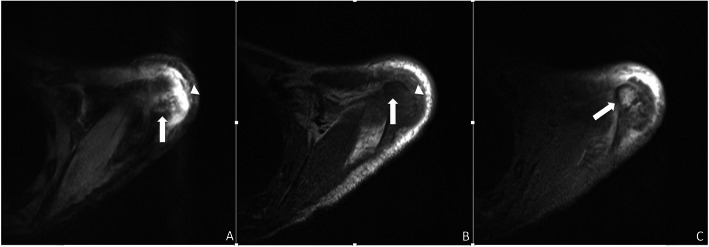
Left shoulder MRI axial images demonstrate signal characteristics consistent with osteomyelitis at the acromial apophysis: hyperintense (brighter) on T2 weighted imaging (a) and hypointense (darker) on T1 weighted imaging (b) with contrast enhancement on post-gadolinium T1 weighted imaging with fat suppression (white arrow c). Cortical disruption (arrowhead b) and an overlying fluid collection (arrowhead a) are also present
